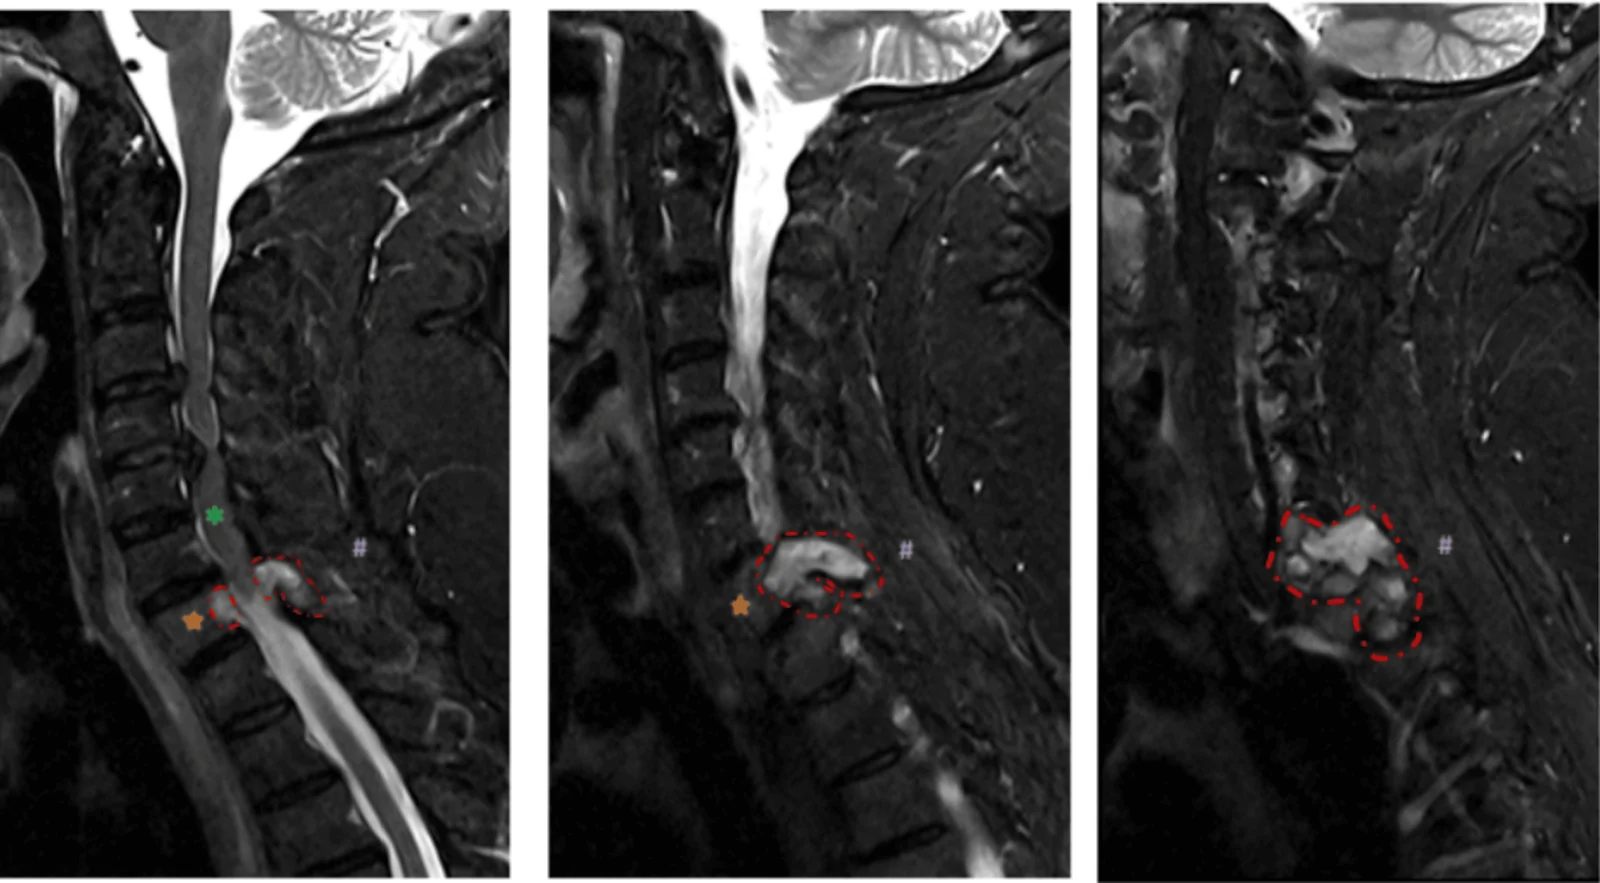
Cervical MRI demonstrating the localization of the FGF23-producing tumor at the C7 vertebral level MRI: magnetic resonance imaging; FGF23: FGF-23: fibroblast growth factor 23 Infiltrative lesion at the C7 vertebra with osseous destruction. The orange and green asterisks delineate the tumor margins, while the red circles indicate the tumor dimensions on different imaging planes
